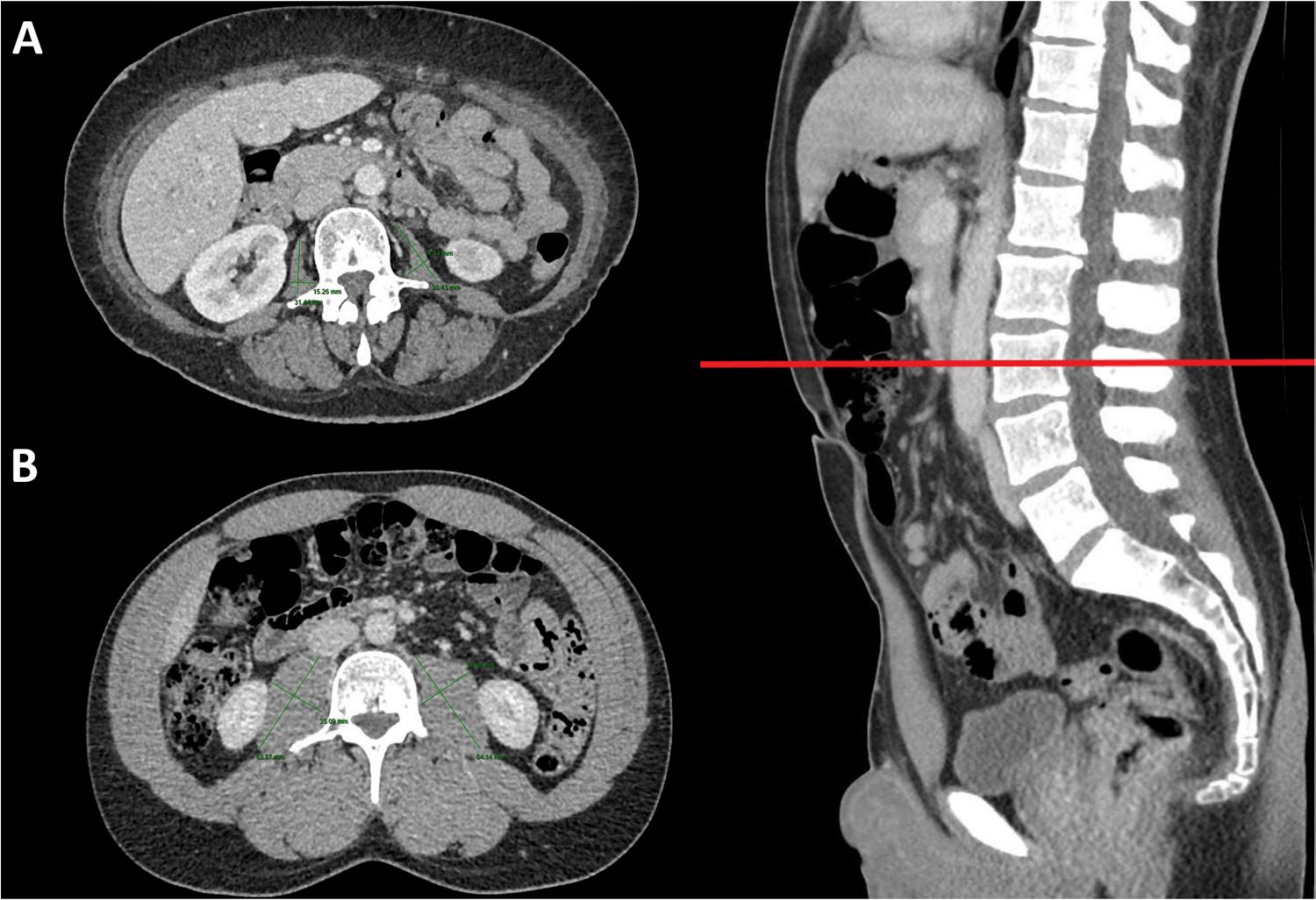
Abdominal computed tomography scans showing the total psoas area measurement in a sarcopenic patient (A) and a non-sarcopenic patient (B) at the third lumbar vertebra
